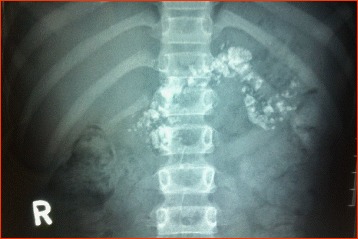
Abdominal X-ray in a patient with FCPD showing pancreatic calculi
